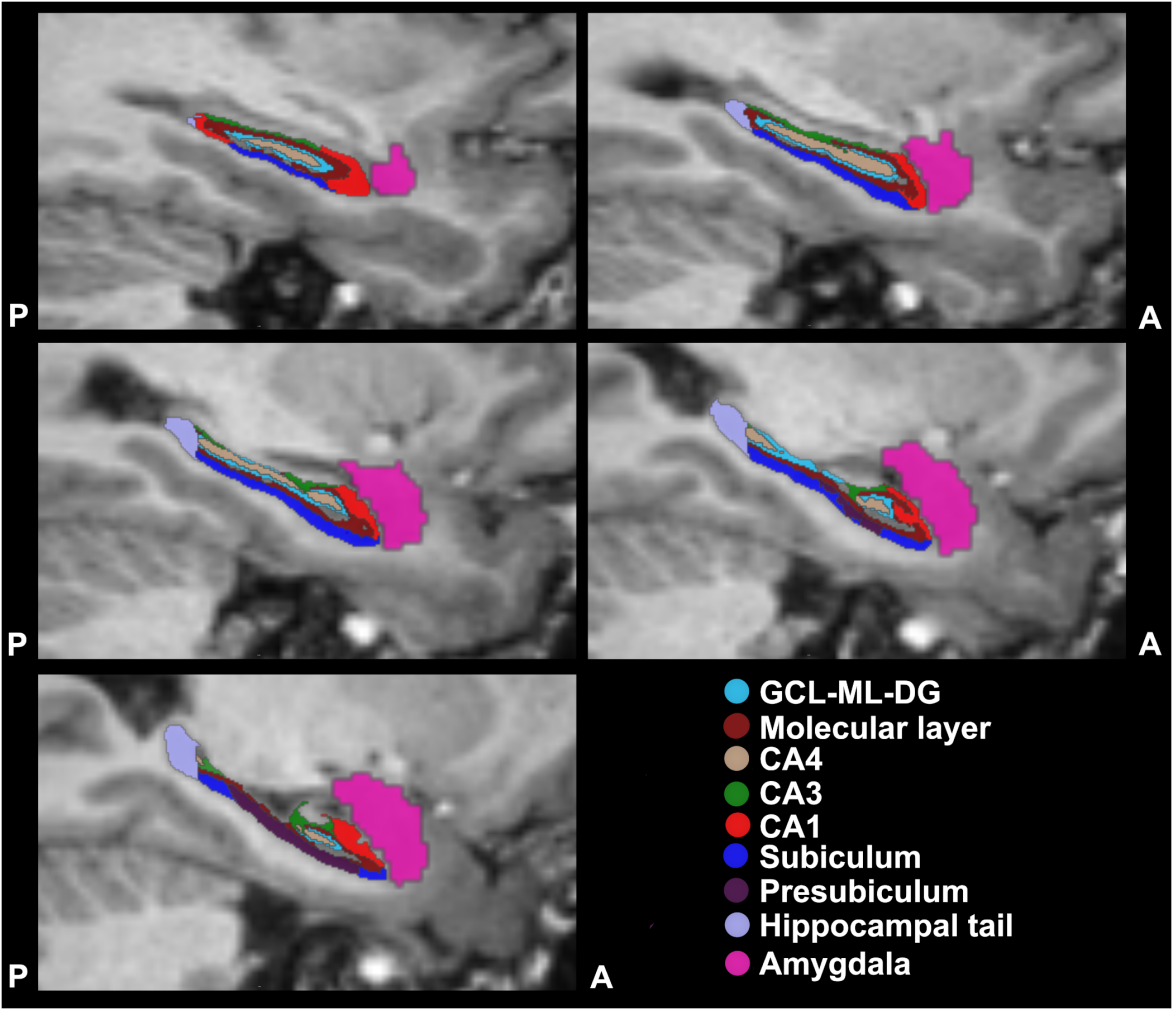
Reconstruction and segmentation of amygdala and hippocampal subfields. Representative T1-weighted images of reconstructed subareas from the left side of the brain. Five slices are shown here in the sagittal plane, in a direction from left to right, i.e. X = -29 to X = - 21 in the Talairach space. The segmentation was based on the ex vivo hippocampal subfield atlas described by Iglesias et al. (84). The different colours indicate different subfield areas. The reconstructed 3D volumes of the different subfields were correlated with the results of the psychological tests. A, anterior; CA, Cornu Ammonis; GCL-ML-DG, granule cell layer and molecular layer of the dentate gyrus; P, posterior.
